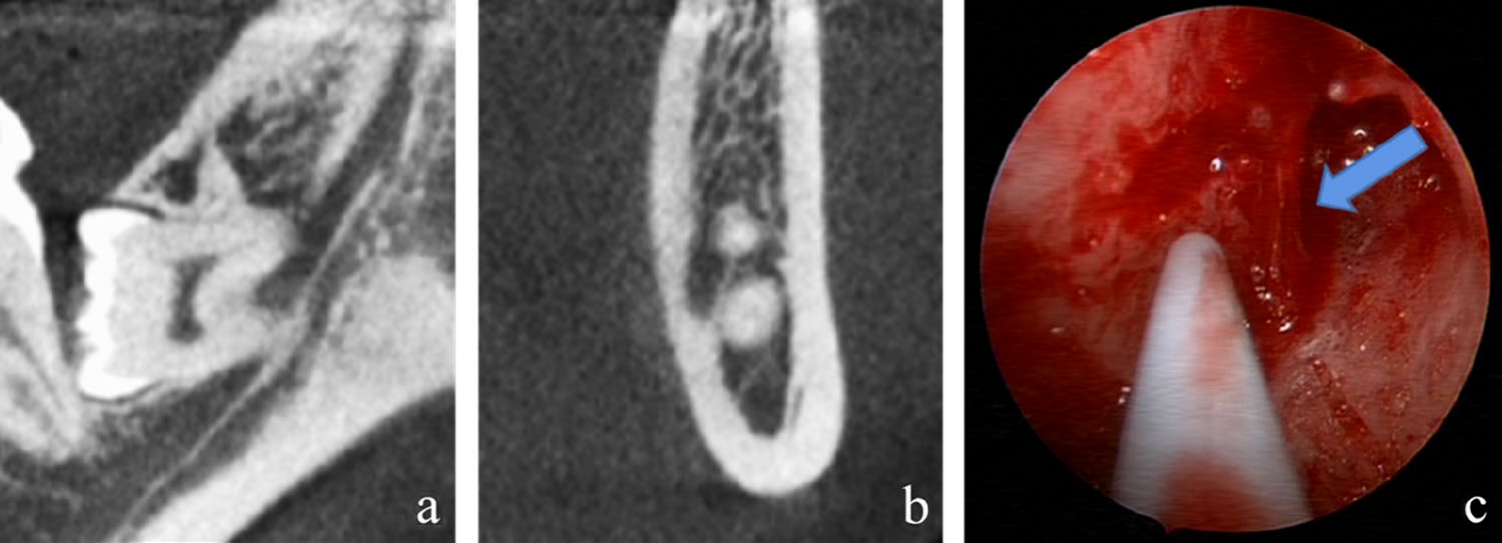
a and b Show the position of the inferior alveolar canal (IAC) to the third molar roots on CBCT images of a patient. The IAC position was categorized into inter-radicular position. c Shows endoscopic view of a possible injured IAN indicated by blue arrow
